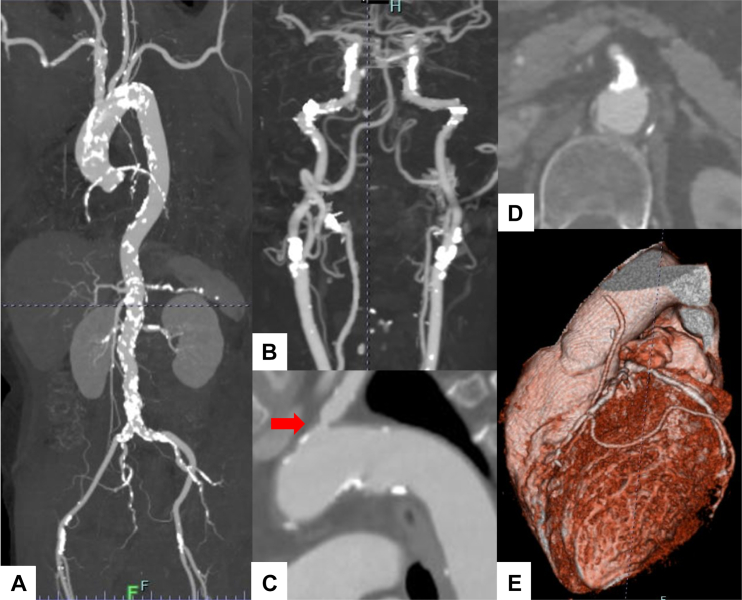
Imaging of Patient 3 Computed tomography revealed widespread calcified stenoses affecting (A and B) the bilateral common and internal carotid arteries, (C) left subclavian artery (red arrow), and (D) superior mesenteric artery. (E) Coronary computed tomography angiography revealed the patency of the left internal thoracic artery graft in the left anterior descending and posterolateral branches.
